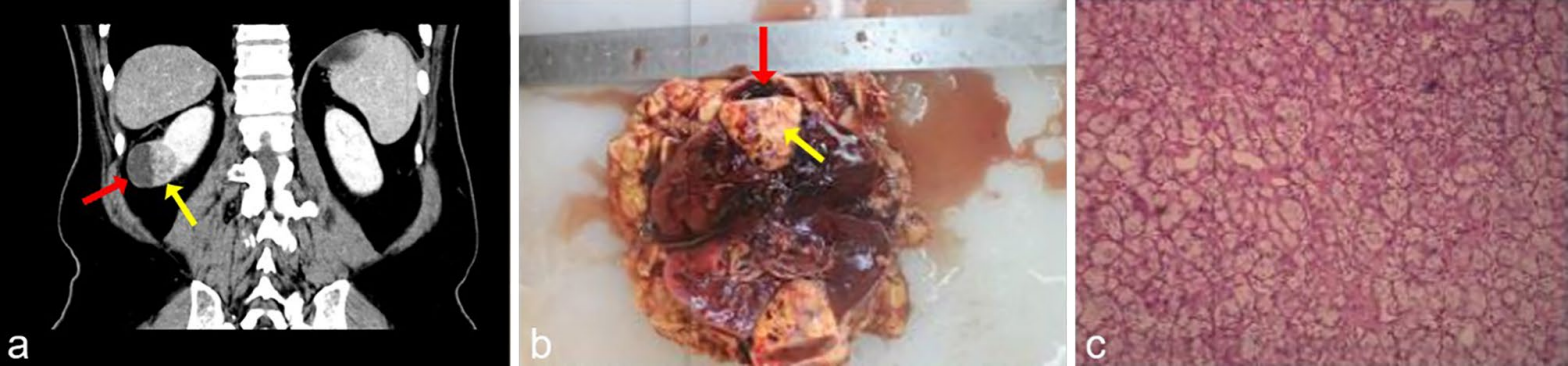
A 60-year-old male with a unilocular cRCC. (a) Postcontrast CT: unenhanced cyst fluid (red arrow) and solid component (yellow arrow). (b,c) Pathology report: Non-necrotic cyst and Fuhrman grade II clear cell carcinoma of the solid component.
